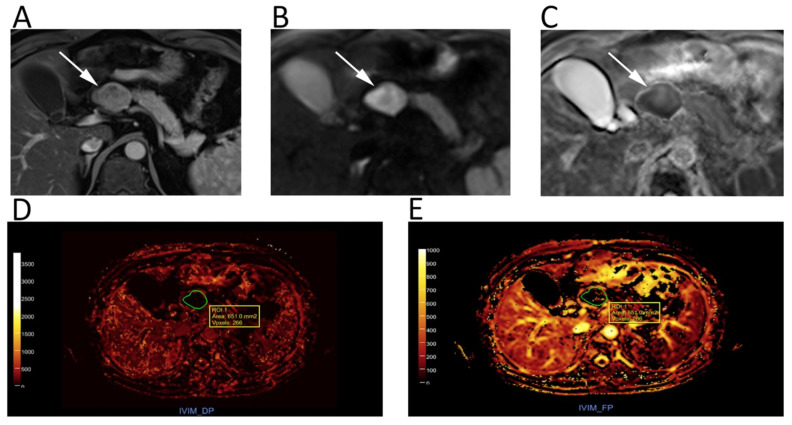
Upper abdomen MRI of a patient with G2 pancreatic neuroendocrine neoplasm. A heterogeneous hypervascular lesion (arrows) with well-defined margins can be seen on the axial arterial phase image (A). The lesion is clearly hyperintense on *b* = 800 DWI (B), with low ADC values on the corresponding ADC map (C). IVIM maps show that the tumor has low values of fast diffusion (D) Dp, but relatively preserved perfusion fraction (E) Fp, consistent with a neuroendocrine neoplasm.
